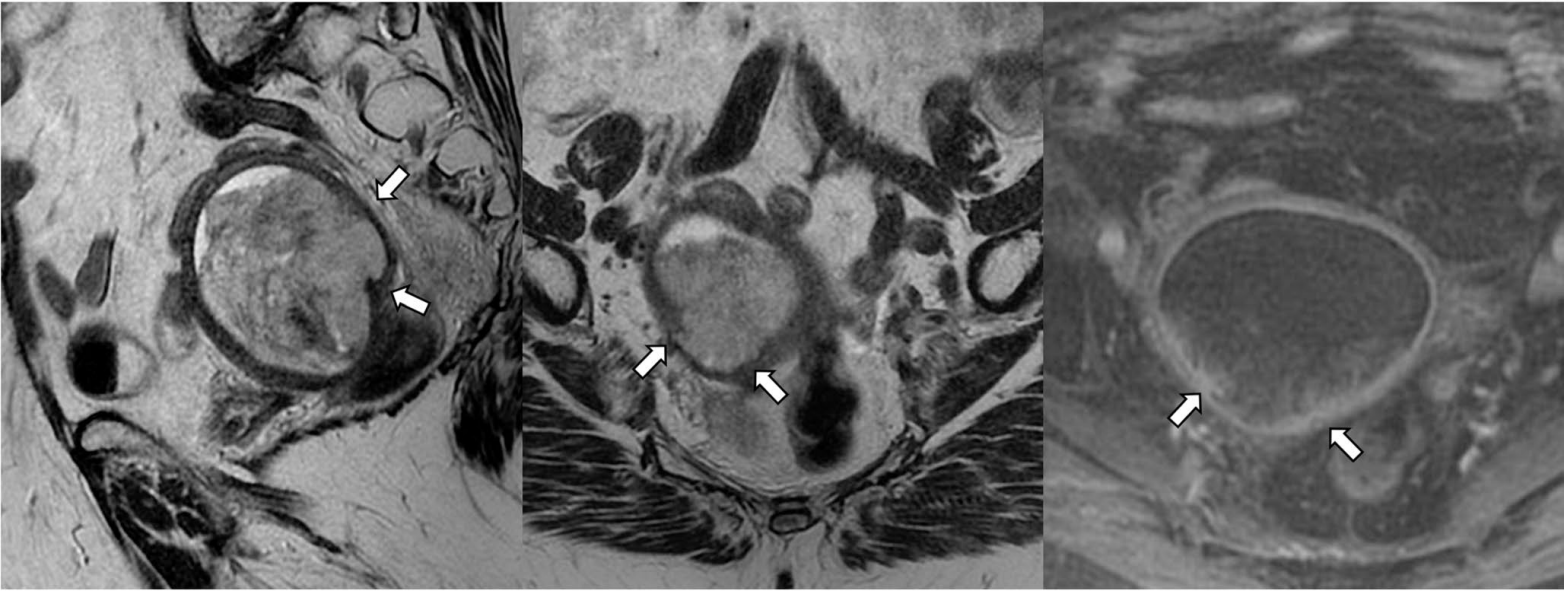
Stage IB grade 2 endometrioid carcinoma, no LVSI in a 60-year-old woman. A Sagittal T2-weighted fast spin-echo (FSE) image (TR/TE: 4500/100 ms; slice thickness: 3 mm) and B axial oblique T2-weighted fast spin-echo (FSE) image (TR/TE: 4500/100 ms; slice thickness: 3 mm) show an endometrial mass with myometrial invasion (arrows). C Axial contrast-enhanced 3D T1-weighted fat-suppressed LAVA sequence (TR/TE: 4.2/2.1 ms; slice thickness: 2 mm; post-gadolinium, delayed phase at 4 min) shows disruption of the junctional zone. Pathology confirmed low grade endometrioid carcinoma involving > 50% of the myometrium
